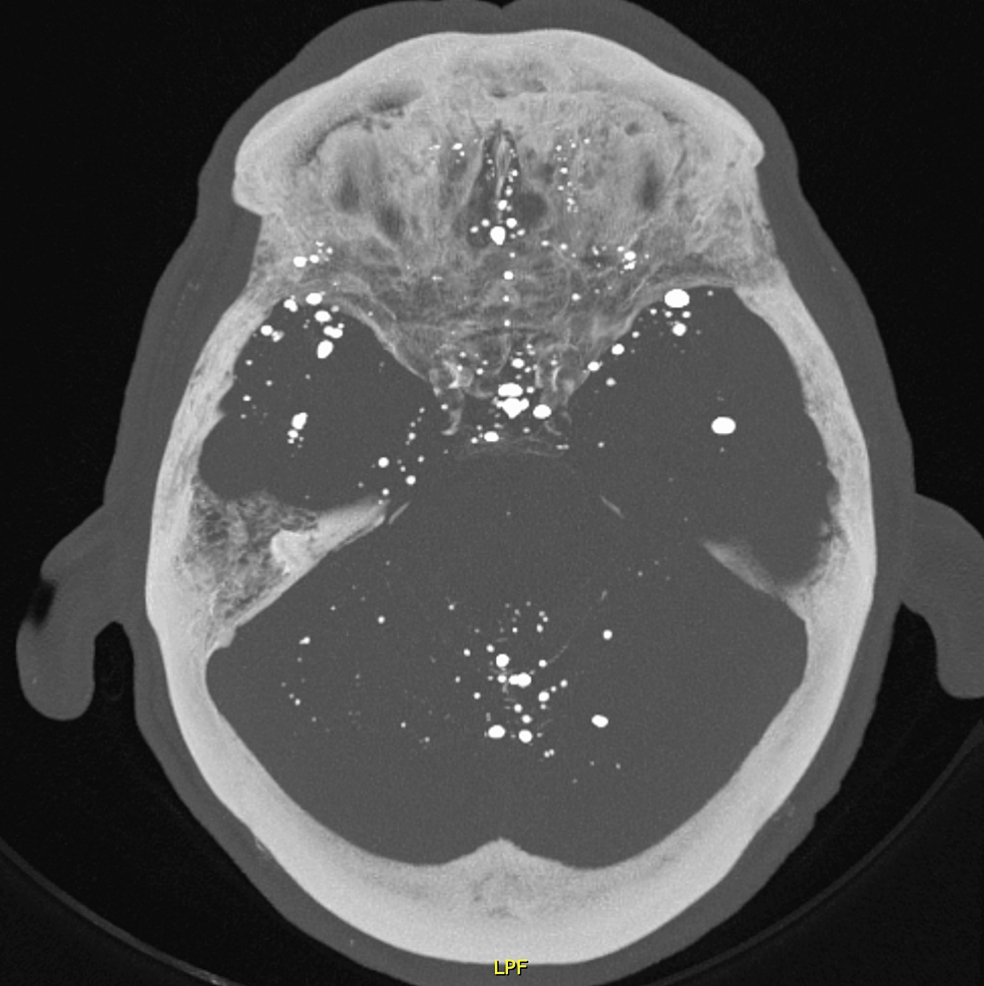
Maximum intensity projection (MIP) of an axial CT scan of the skull. MIP of an axial CT-scan image showing innumerable hyperdense droplets, situated in the temporal and frontal areas as well as within the spaces between the folia of the cerebellum.
